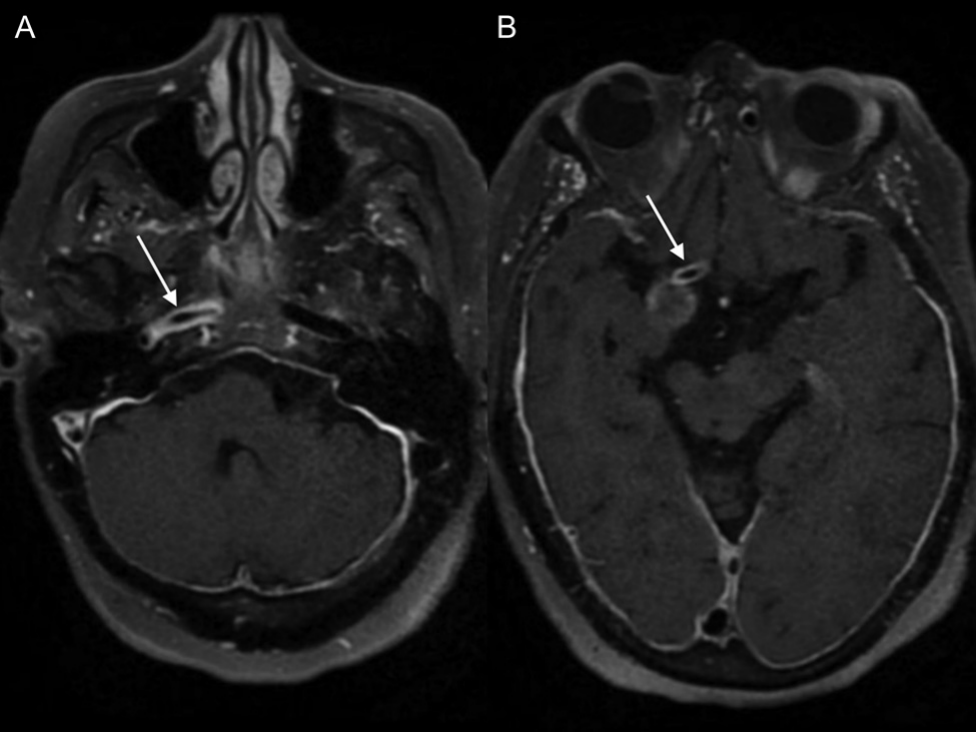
Postgadolinium axial vessel wall magnetic resonance images of the petrous (
